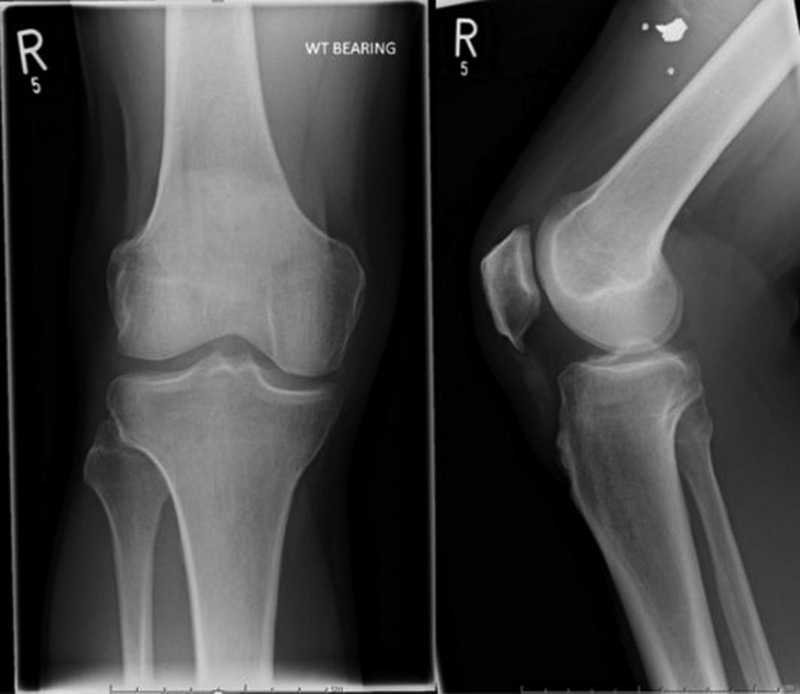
Plain anteroposterior and lateral radiographs.
